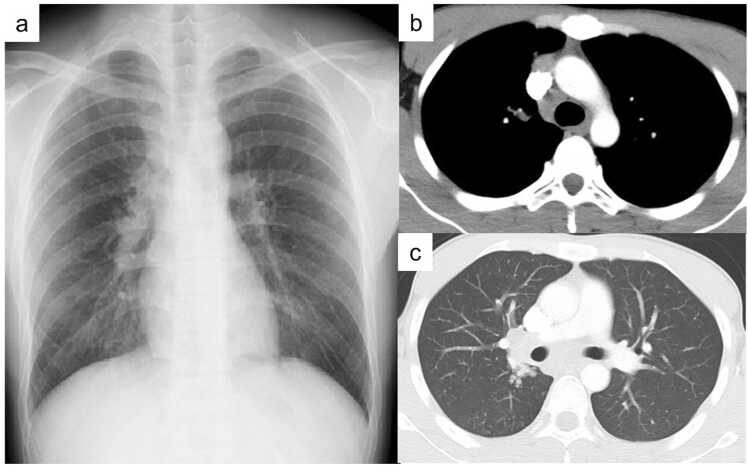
Chest X-ray showing mediastinal widening at presentation (a). Chest CT before treatment demonstrating enlarged mediastinal lymph nodes (b) and pericardial effusion (c).
